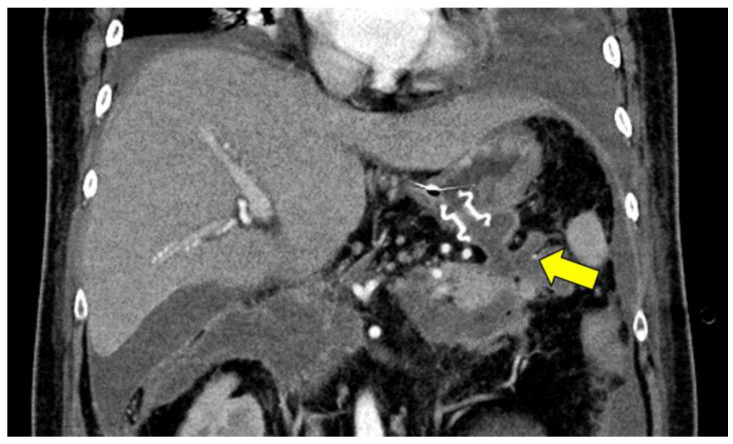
CT after 40 days of admission. WON is observed around the pancreas. The small vessels are observed in the pancreatic tail WON cavity (arrow). LAMS is placed into the pancreatic tail side WON cavity.
